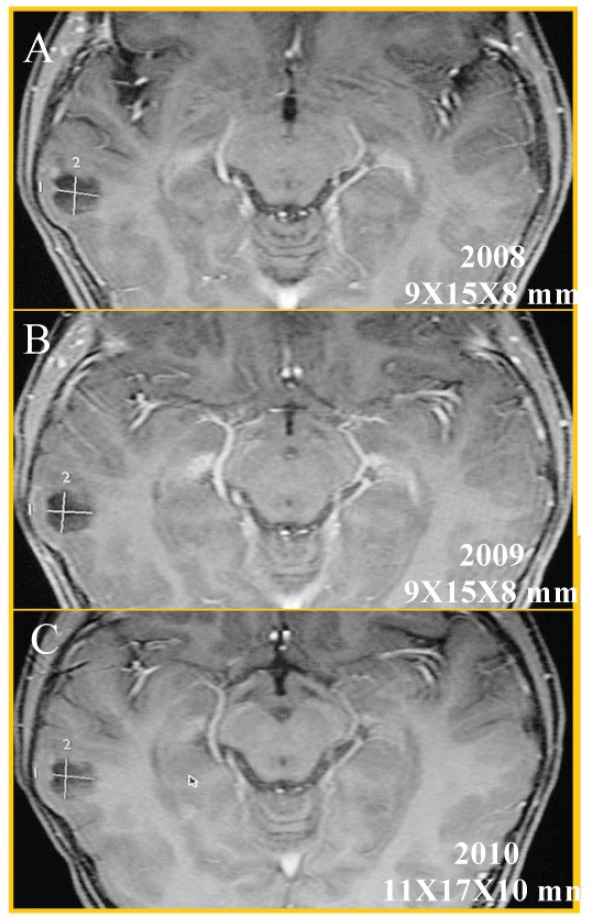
Axial T1w MRI + gadolinium, follow-up of two and a half years; (A) 2008, (B) 2009, and (C) 2010, which showed the temporal injury (white measurements) had grown 2 mm. This lesion measured 9 × 15 × 8 mm and currently measures 11 × 17 × 10 mm along its longest axis.
